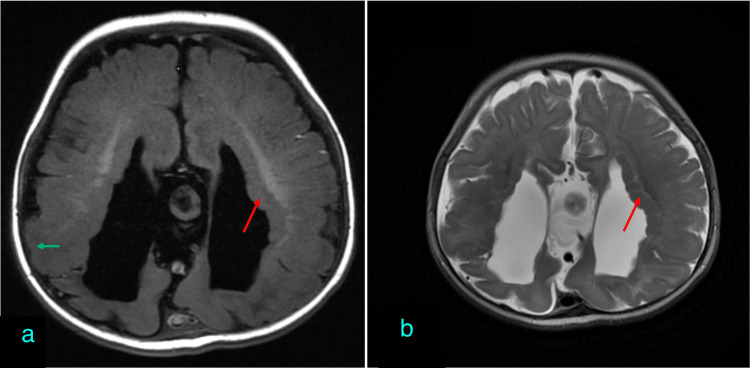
Corpus callosum agenesis with cortical malformation. (a) Axial T1-weighted imaging showing the absence of corpus callosum with diffuse periventricular gray matter heterotopia (red arrow). Gyral abnormality in the form of focal pachygyria in the right parietal lobe (green arrow). (b) Axial T2-weighted imaging showing the absence of corpus callosum with diffuse periventricular gray matter heterotopia (red arrow).
